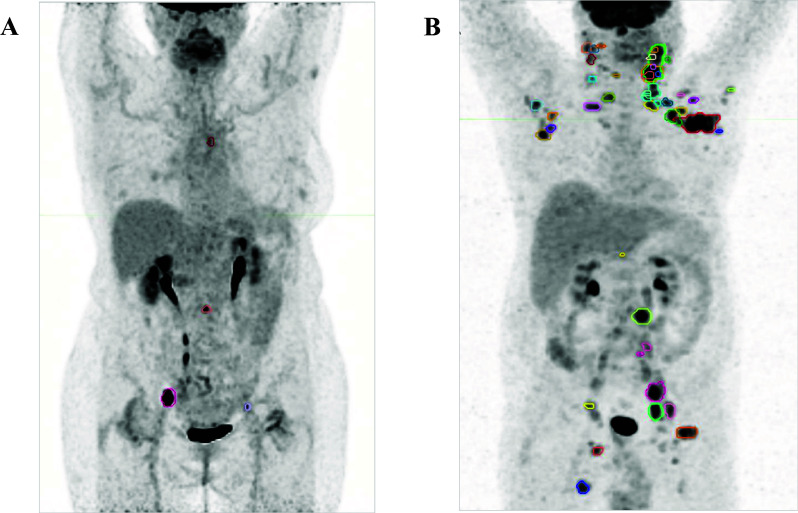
Total metabolic tumour volume (TMTV) and whole-body total lesion glycolysis (WB-TLG) delineated at baseline FDG PET/CT using an automatic whole-body segmentation software. A. A 68-year-old female patient with low tumour burden follicular lymphoma (FL): grading 2, stage IIA, Follicular Lymphoma International Prognostic Index (FLIPI) = 1. She had been on WW for 79 months, when she started R-COMP for disease progression. TMTV = 7.3, WB-TLG = 44.6; B. A 58-year-old male patient with low tumour burden FL: grading 2, stage IVA, FLIPI = 2. He started treatment with six cycles R-CHOP + 2 × Rituximab maintenance for disease progression 9.4 months after diagnosis. TMTV = 143.8 and WB-TLG = 657.1
